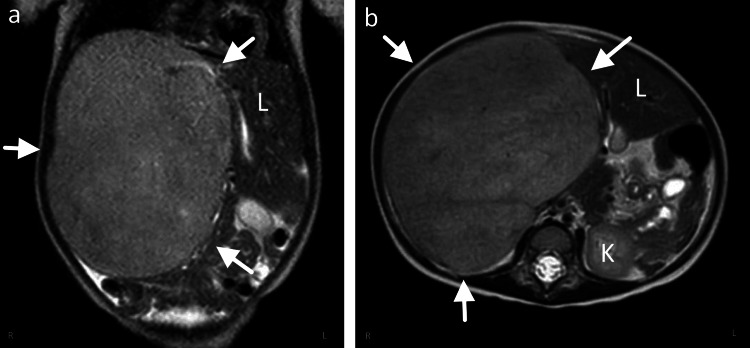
MRI abdomen coronal (a) and axial (b) views of 14.1 x 11.5 x 14.5 cm solid mass involving the anterior and posterior segments of the right hepatic lobe L: normal liver; K: kidney; arrows: boundaries of hepatic neoplasm
